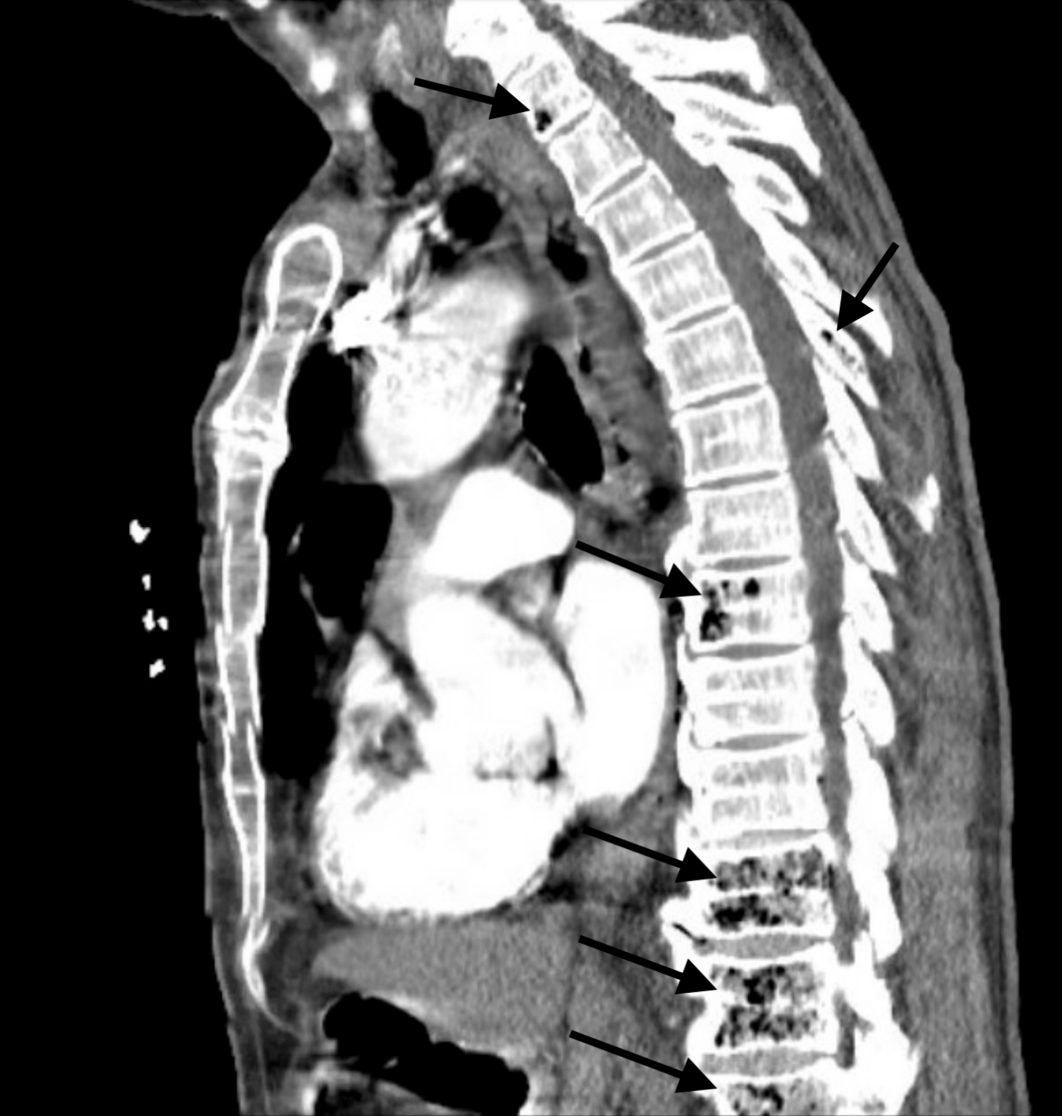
Emphysematous osteomyelitis of the thoracic and lumbar spine on CT. Sagittal CT angio chest in an 80-year-old Caucasian man presenting with worsening generalized weakness, dyspnea, and tachycardia with black arrows showing diffuse intraosseous gas within T1, T8, T11, T12, and L1 vertebrae as well as the T5 spinous process.
